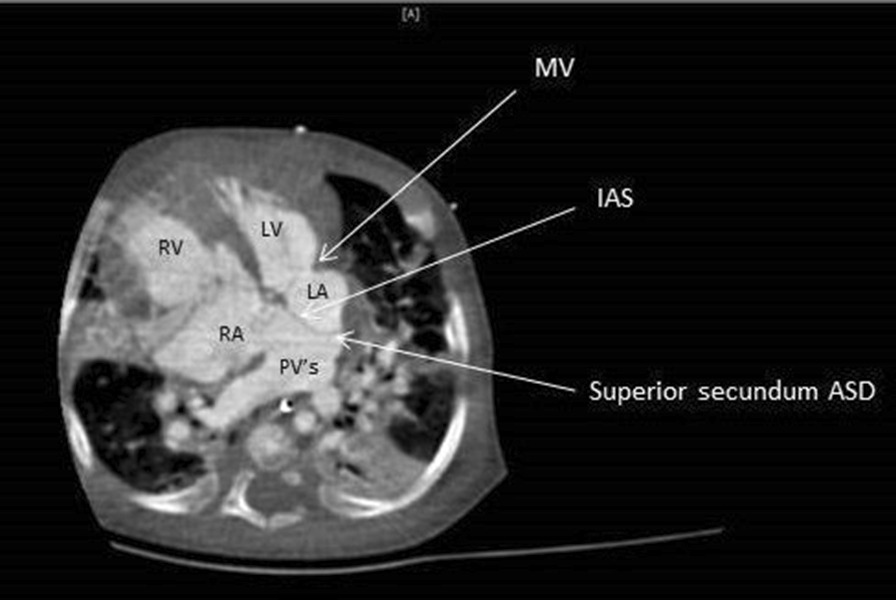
CT angiogram showing TAPVR (MV—mitral valve, IAS—inter atrial septum, ASD—atrial septal defect, PV—pulmonary veins opening in to the RA, RV—right ventricle, LV—left ventricle, RA—right atrium, LA—left atrium)
